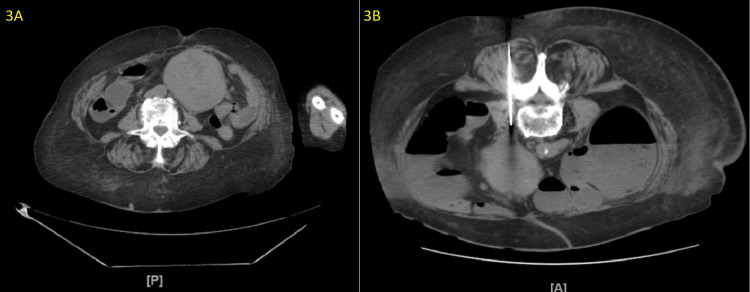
CT of the abdomen. A: CT of the abdomen and pelvis without contrast shows a 6.5 cm × 7.0 cm well-marginated mesenteric mass of the left paramedian abdomen. B: Biopsy of the mesenteric mass of the left paramedian abdomen without infiltration of the adjacent fat planes.
